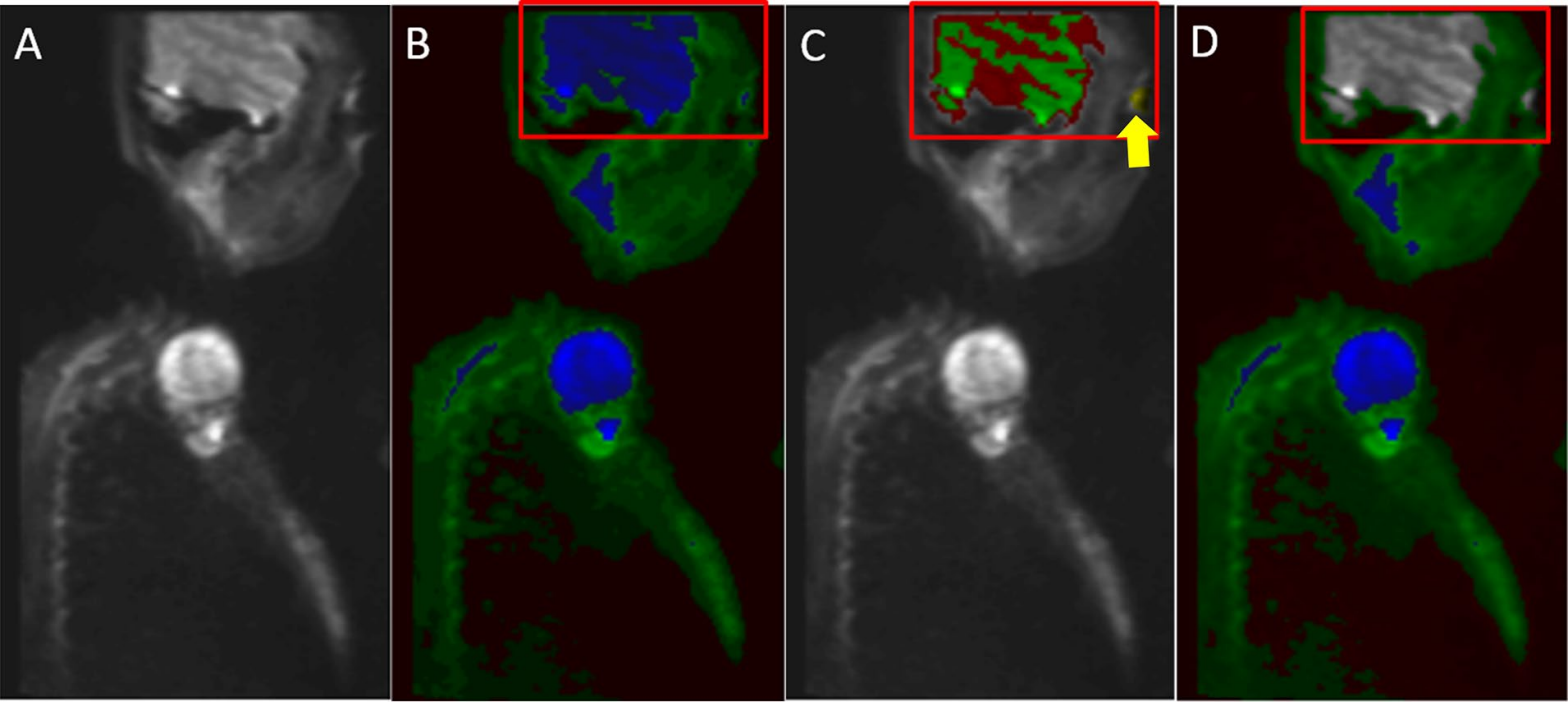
Representative normalized sagittal images of cervical DWI MRI: (A) b = 0 DWI image (unclassified); (B) Initial Classified Object Map based on the MSNN model described in Fig. 1; normal tissue (green) and tumor (blue); (C) Misclassified structures such as Brain (red) and Orbits (yellow arrow) which require exclusion from tumor volume; and (D) Quality control corrected normal (green) and tumor (blue).
